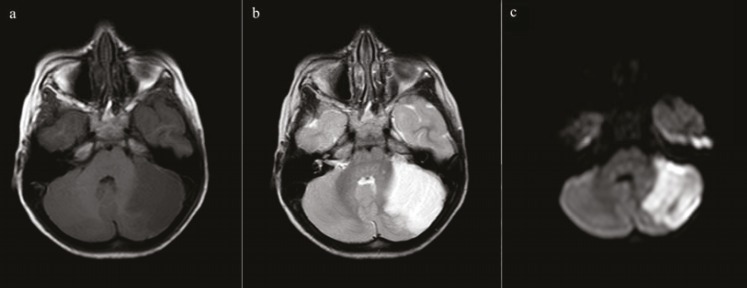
MRI and DWI show an infarction at the superior cerebellar artery territory in the left cerebellar hemisphere. The Infarction is seen as hypointensity on T1 weighted images (a), hyperintensity on T2 weighted images (b), hyperintensity suitable for restricted diffusion on DW images
